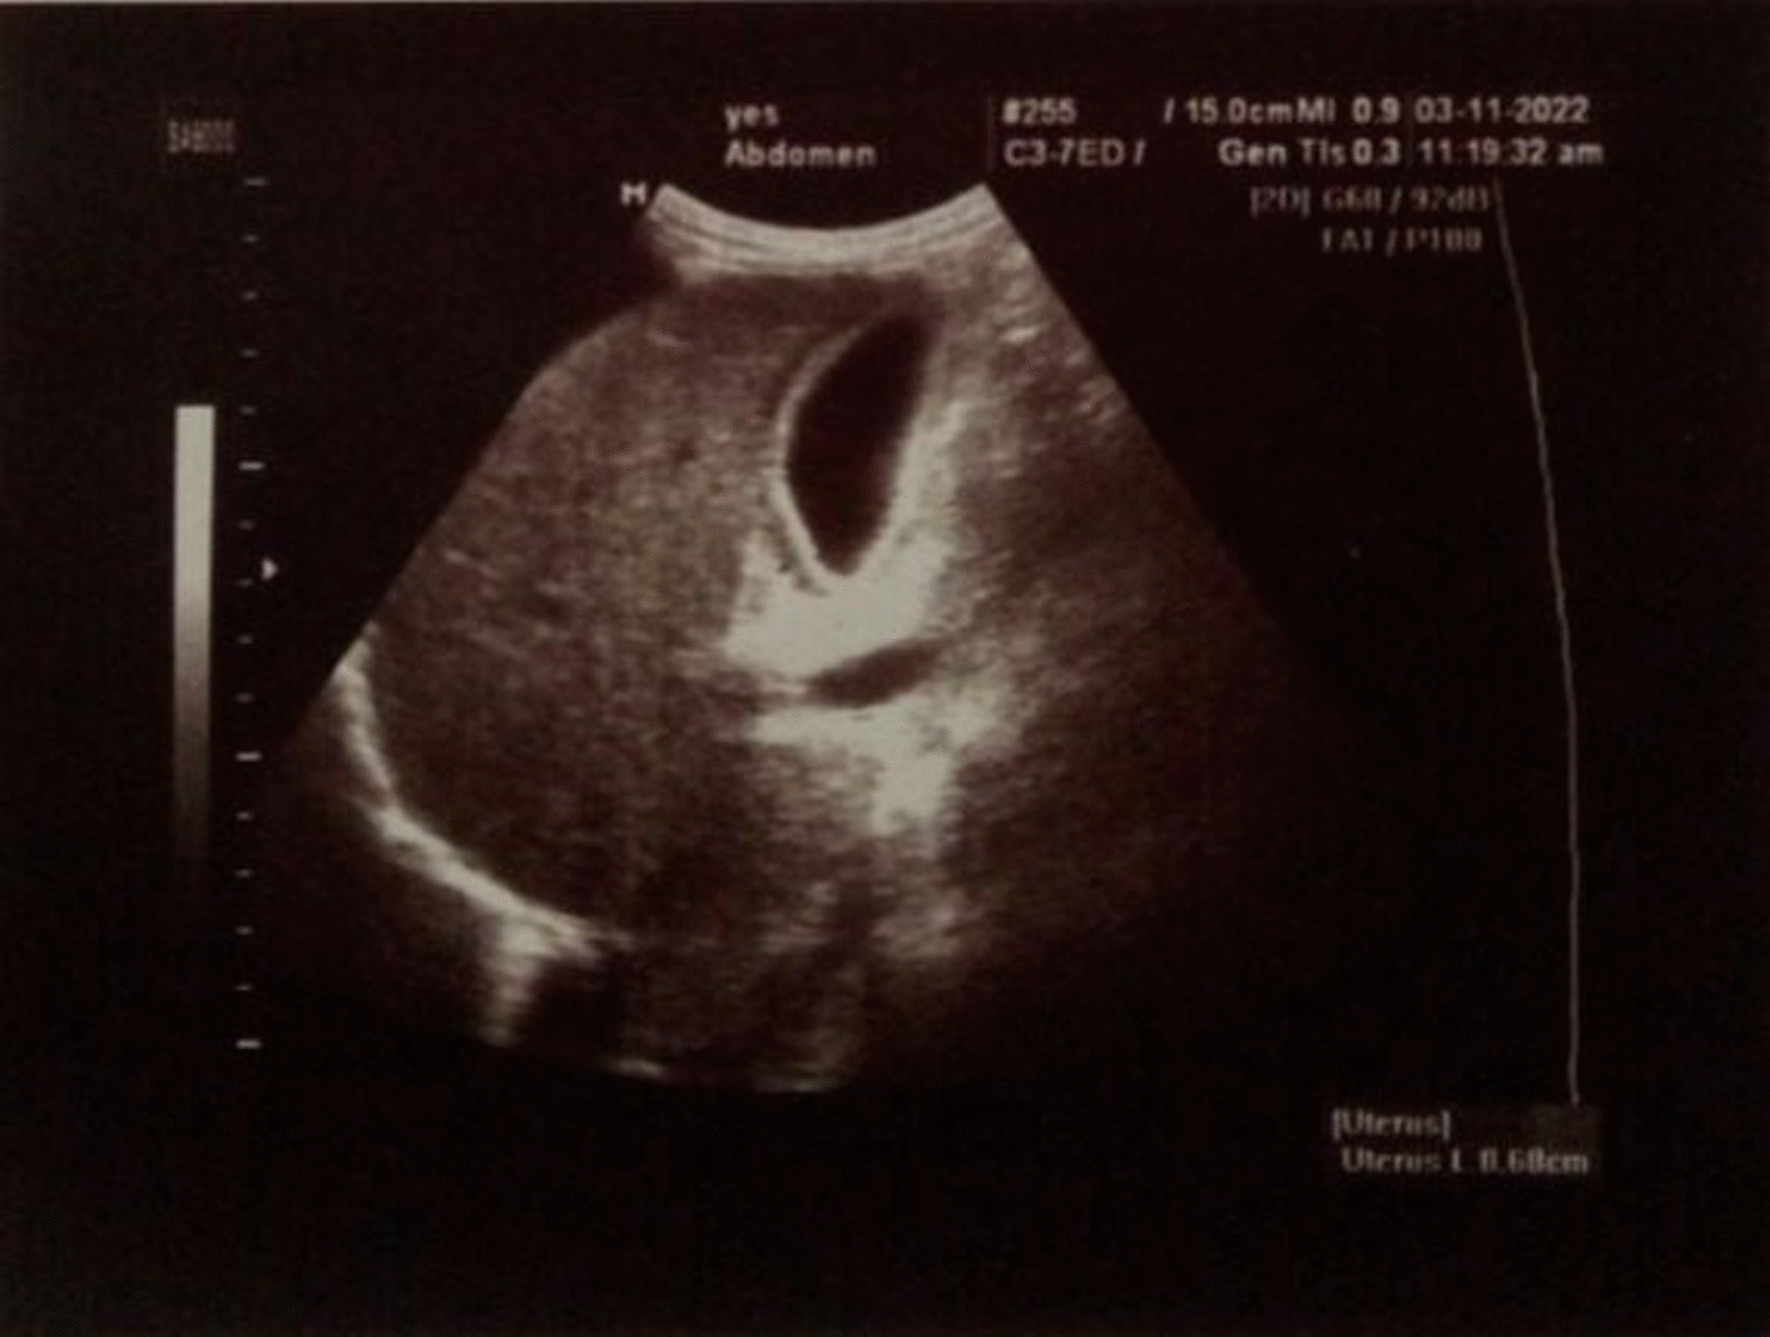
Thickening of the gallbladder wall and edematous pericholecystic area in abdominal ultrasonography
